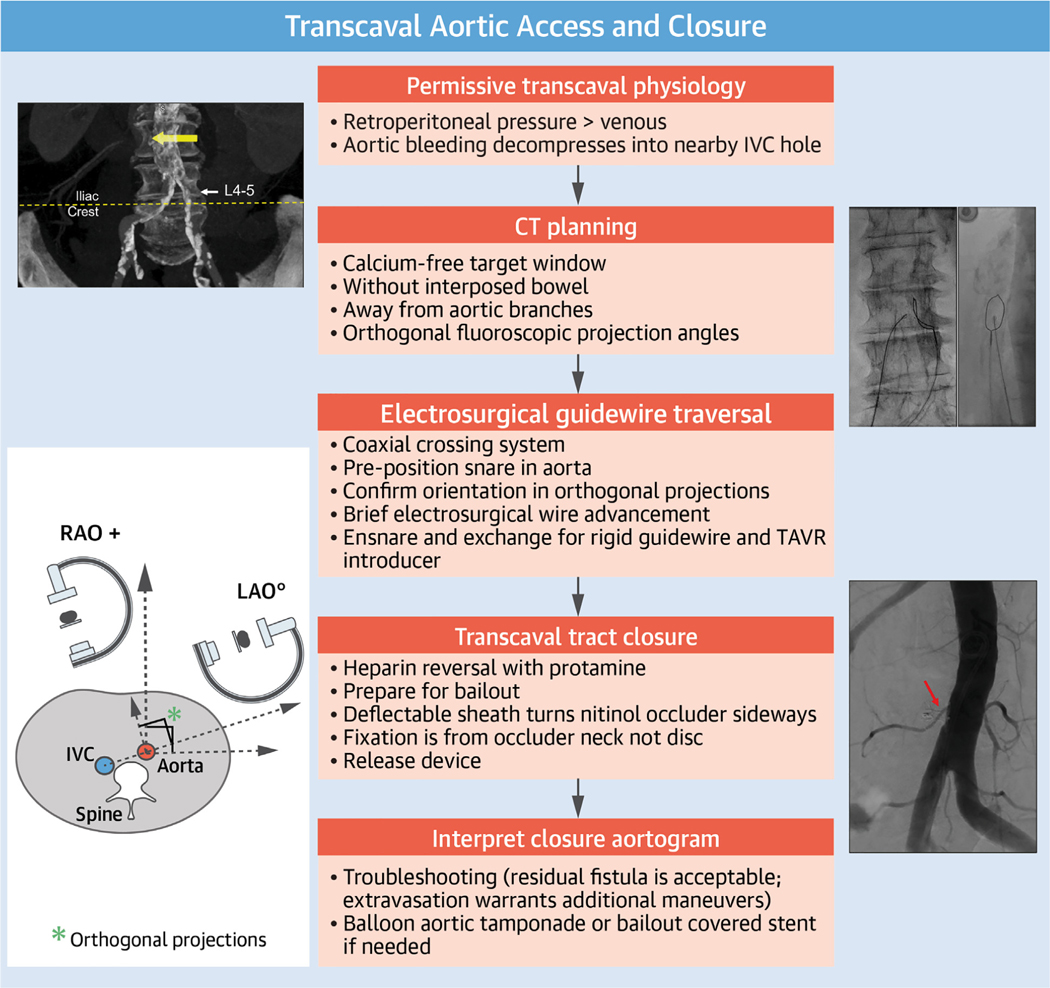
Transcaval Aortic Access CT = computed tomographic; IVC = inferior vena cava; LAO = left anterior oblique; RAO = right anterior oblique; TAVR = transcatheter aortic valve replacement.
